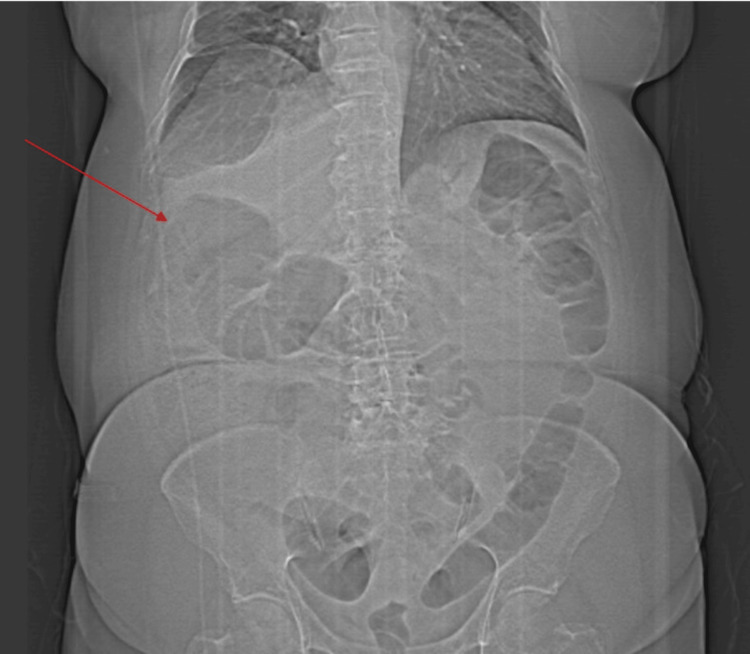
Abdominal X-ray demonstrates "coffee-bean sign"; the cecal volvulus is highlighted (red arrow).
